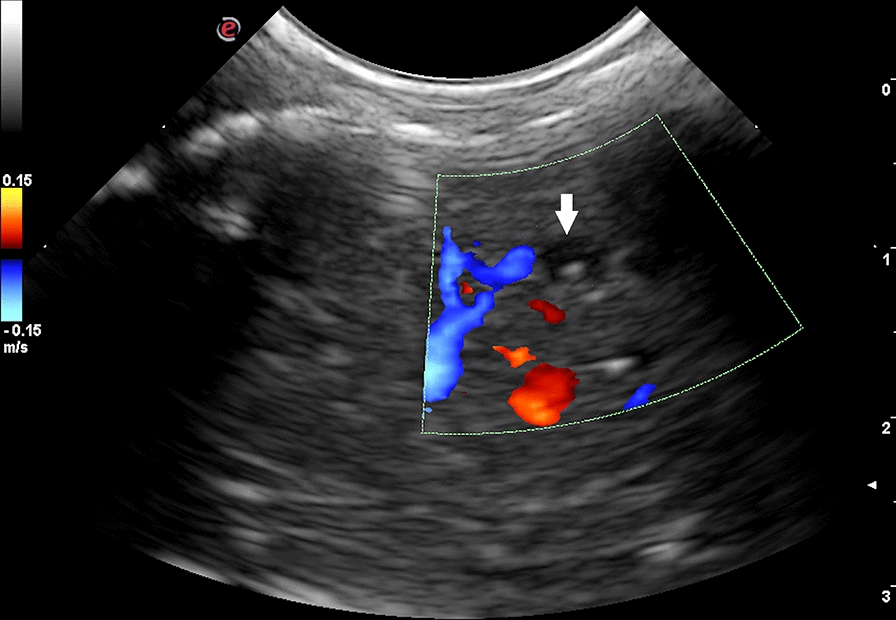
Color Doppler examination of the liver showing an intraparenchymal cyst (arrow)
